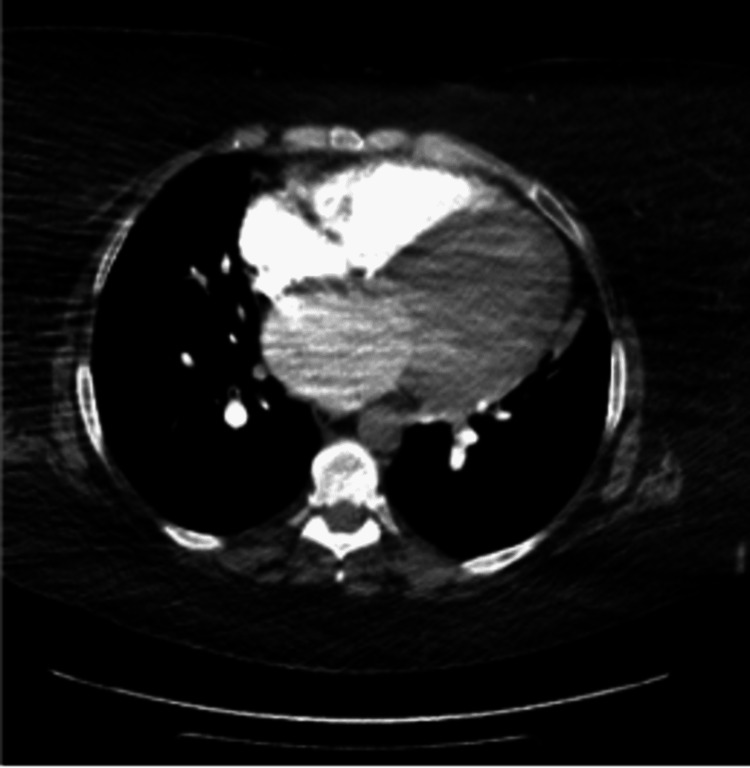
Computed tomography angiography of the chest showing interstitial edema, mild bibasilar atelectasis, and cardiomegaly.
